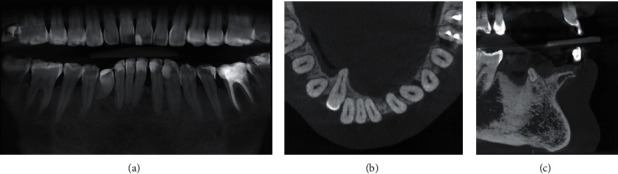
CBCT initial images: coronal (a); axial (b); and sagittal (c) sections.
